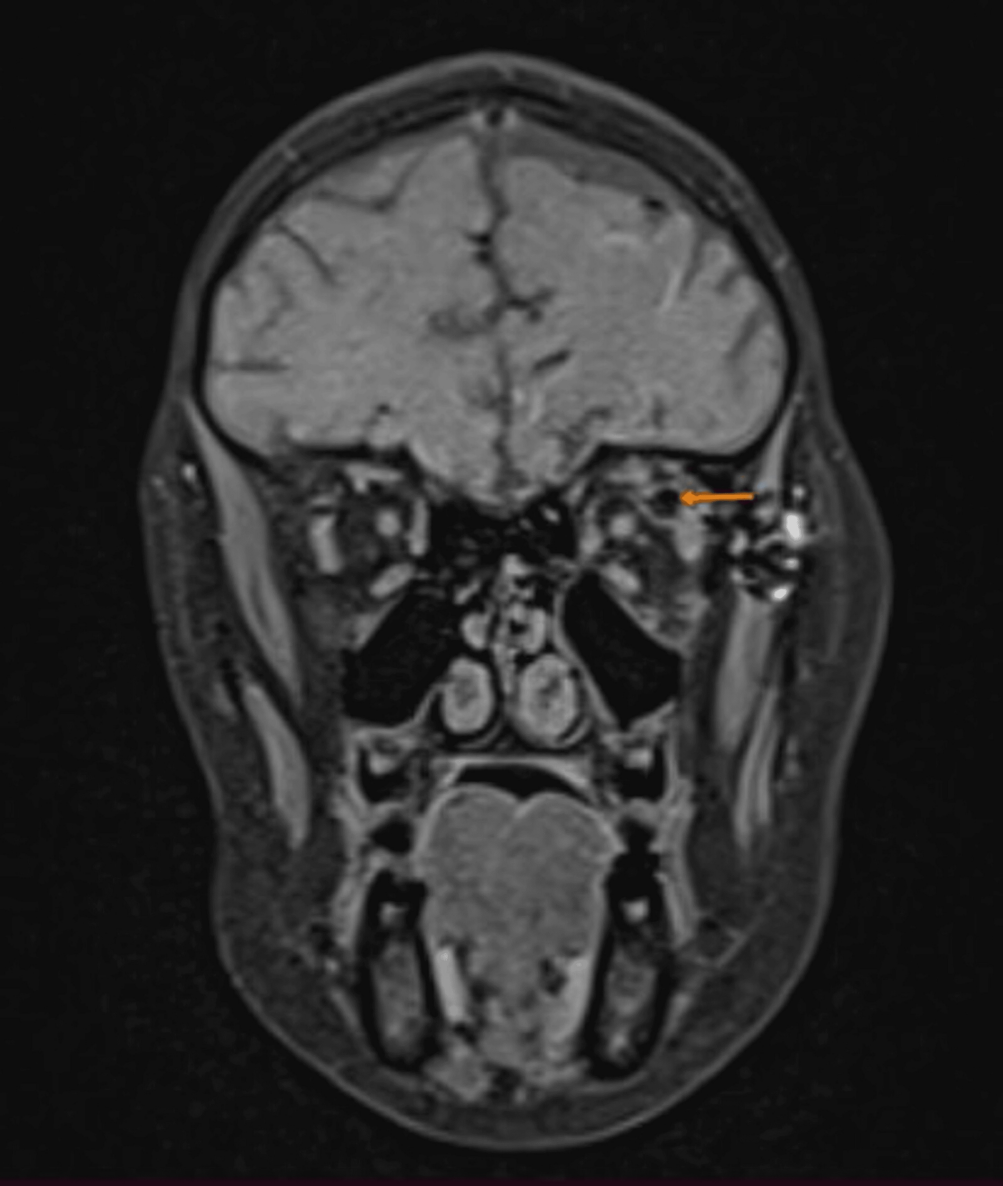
Coronal T1 fat-saturated MRI section showing signal suppression from an intraorbital mass in the left superolateral orbital wall, with hypersignal on T1 and T2 (orange arrow). MRI, magnetic resonance imaging
